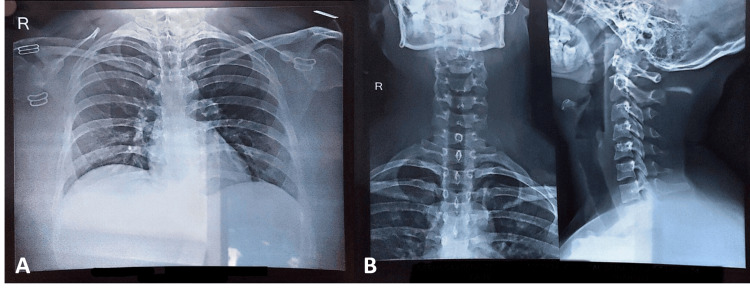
X-rays of (A) chest (posteroanterior view), (B) cervical spine (anteroposterior and lateral views) showing normal observations
